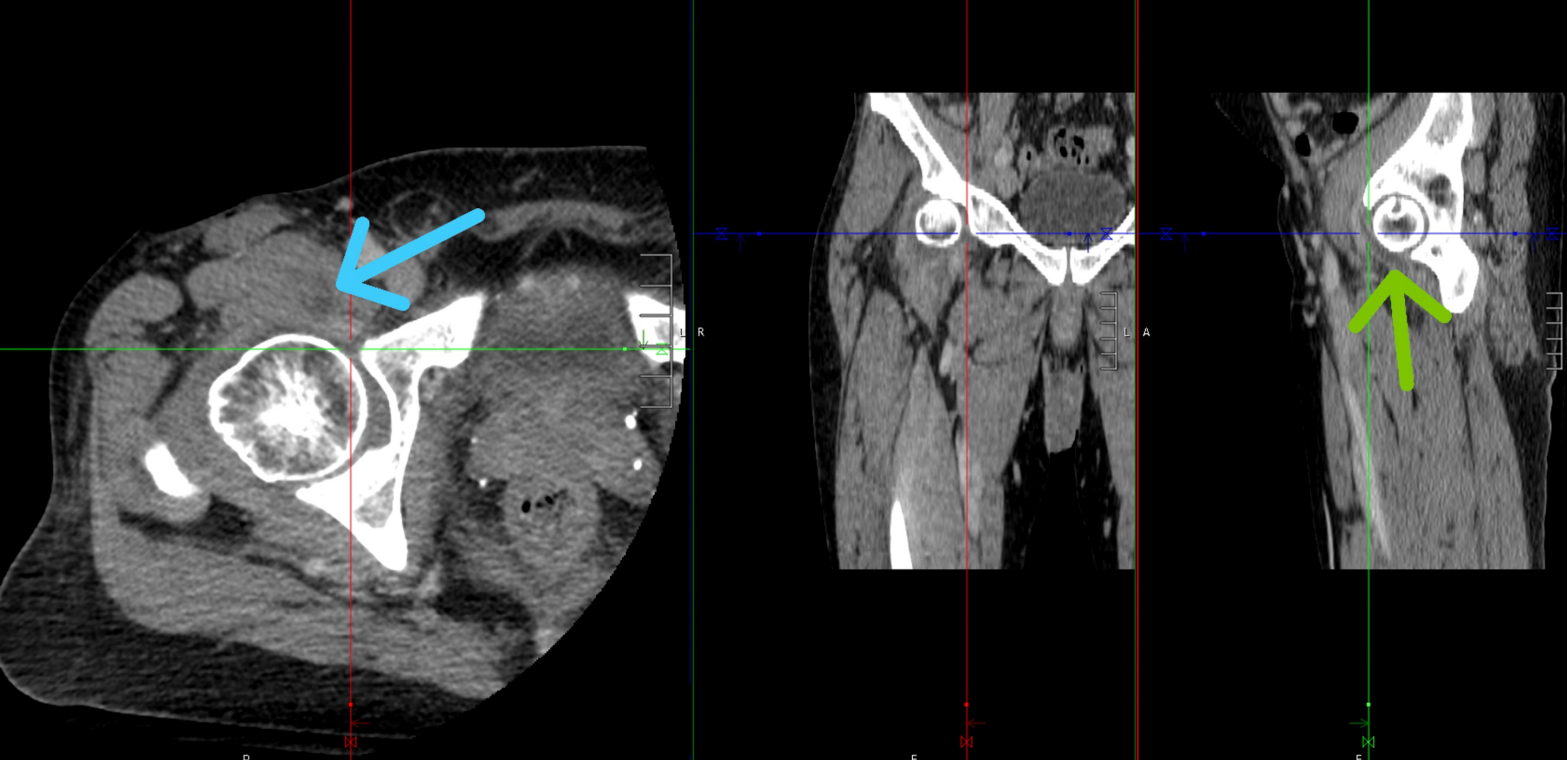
CT of Right Hip The blue arrow denotes a region of fluid in the musculature from the arthrocentesis anesthetic. The green arrow and the red, green, and blue horizontal and vertical triangulation lines pinpoint the small effusion around the femoral head. CT: Computed Tomography
